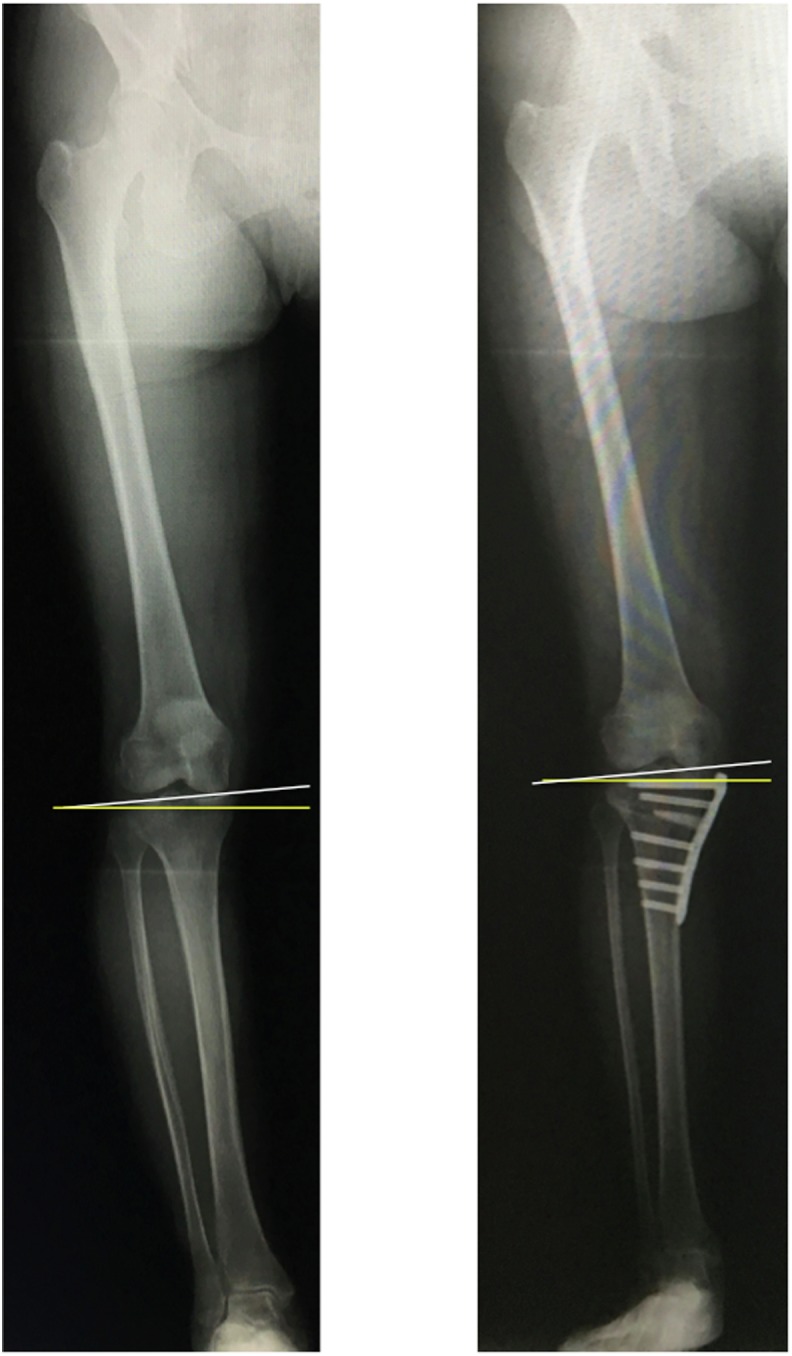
Joint line obliquity. Single-limb standing anteroposterior radiograph of a lower extremity. JLO was defined as the angle between the line parallel to the ground (yellow line) and the articular surface of the proximal tibia (white line). Medial inclination was defined as “+” and lateral inclination was defined as “–”.
